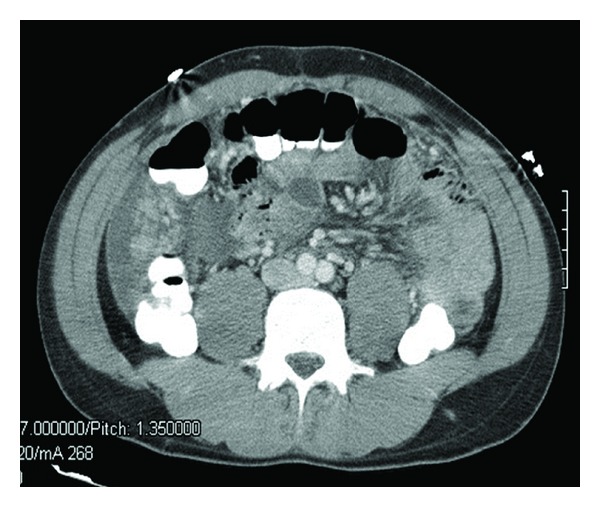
CT scan of the abdomen and pelvis showing ascites with diffuse nodularity of the omentum and peritoneal lining.
